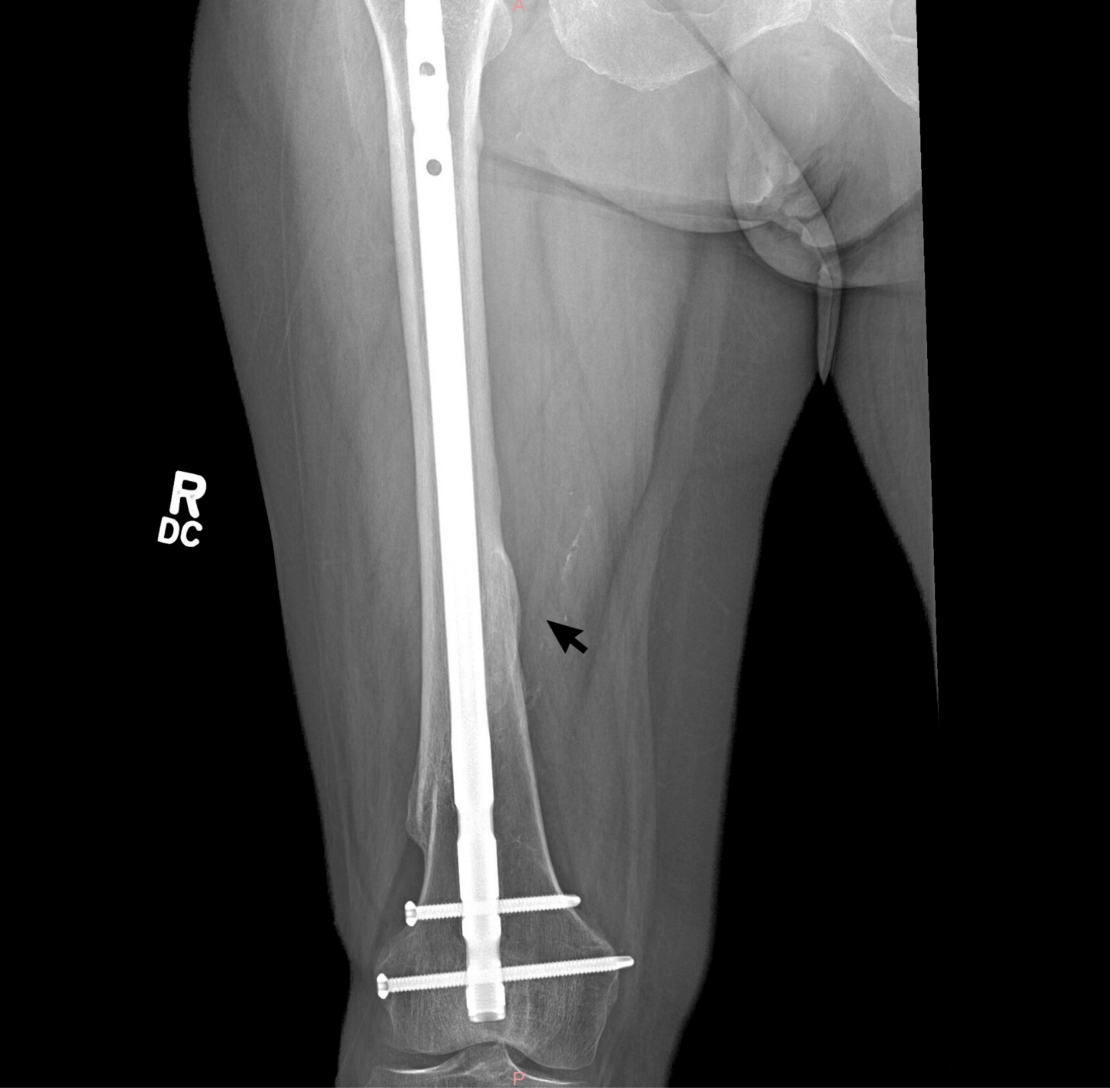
X-ray imaging showcasing healed fracture of the femur with hardware in-situ
